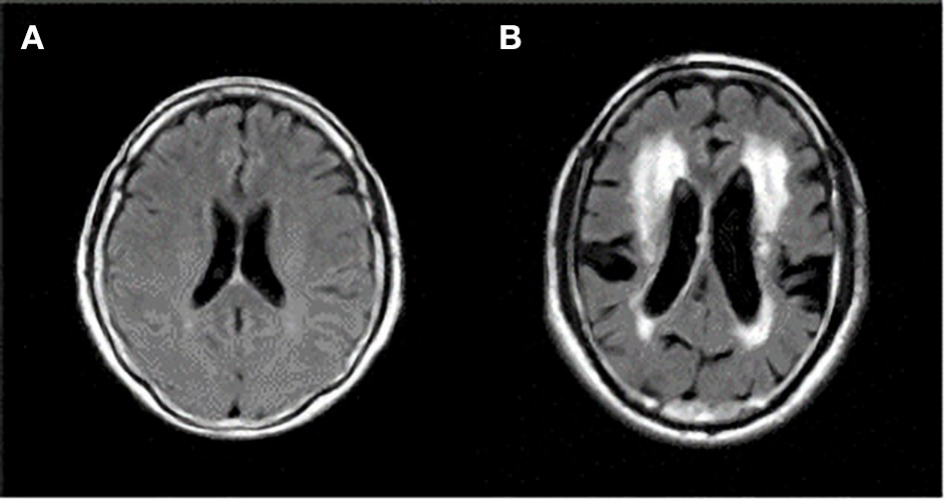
Axial magnetic resonance imaging (MRI) sections of two different brain features of healthy elderly individuals, both 70 years of age, who can drive normally. (A) Minimum of brain atrophy (BA) and leukoaraiosis (LA). (B) Larger BA with enlarged ventricles and sulci, and larger LA around ventricles. The corresponding author owns the copyright of these two MRIs.
